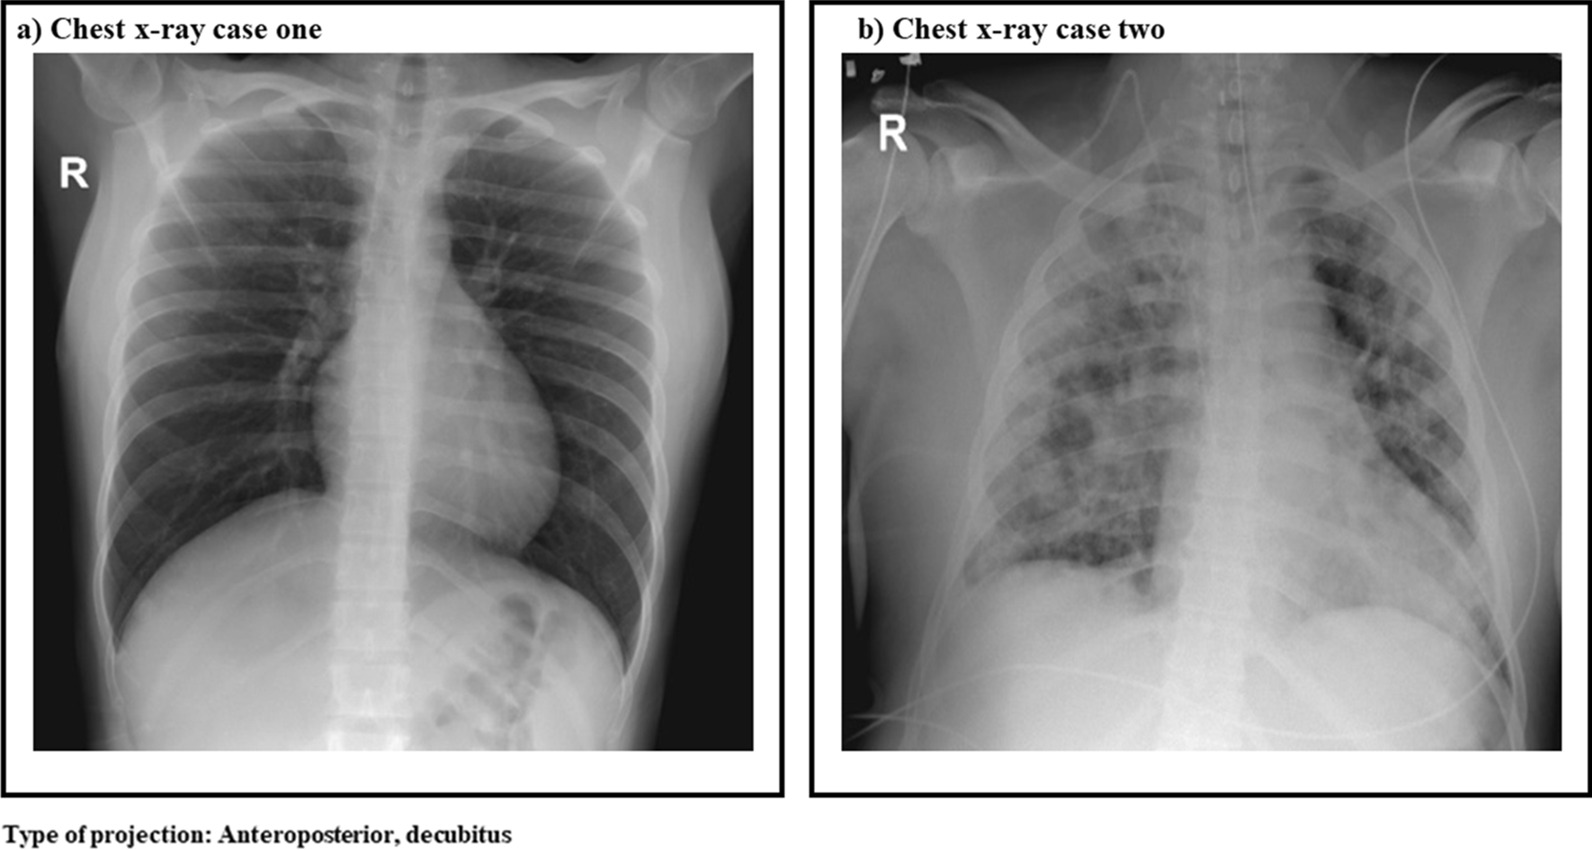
X-ray images of each case
